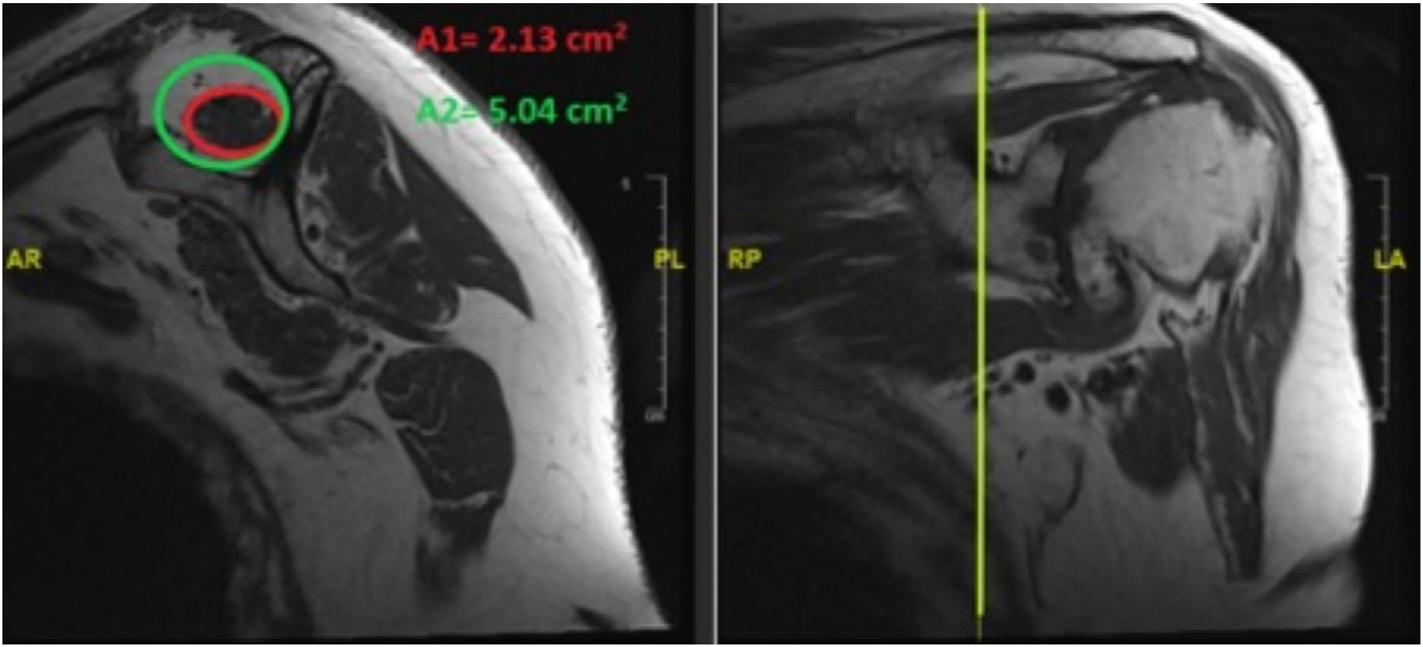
Calculation of occupation ratio using the cross-sectional area of the supraspinatus muscle and its fossa on a sagittal MRI image.
